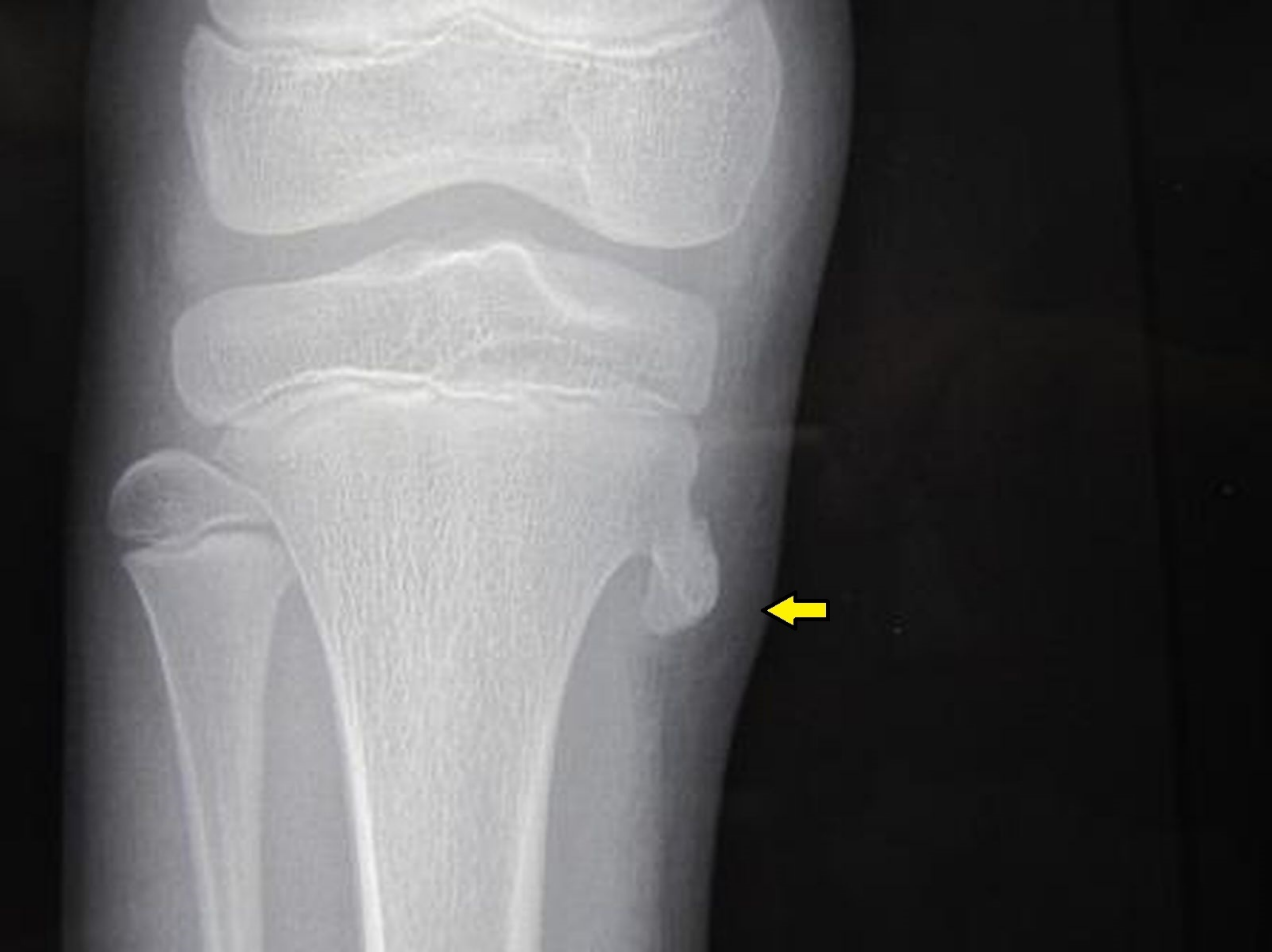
Case 3: Plain radiograph of the right knee—anteroposterior view Presence of a bony outgrowth (yellow arrow) from the medial aspect of right proximal tibial metaphysis suggestive of an osteochondroma.
